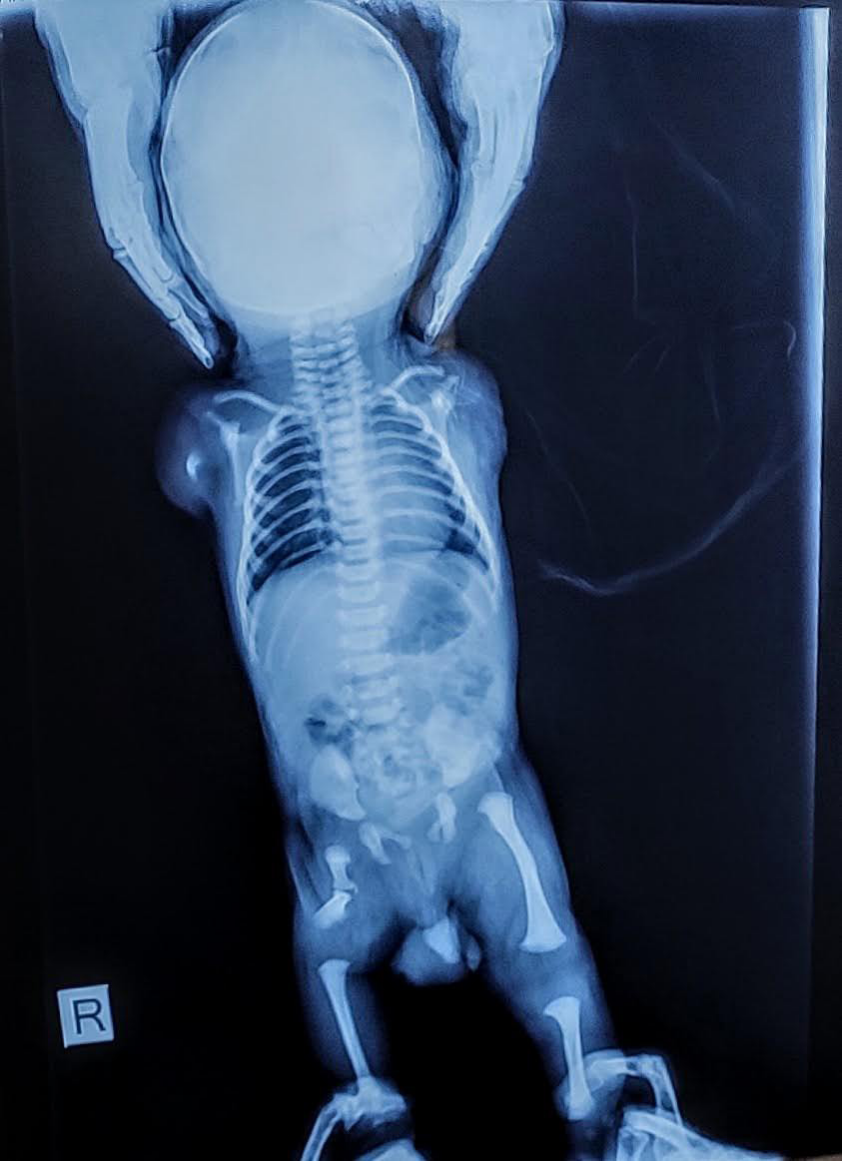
The X-ray of the baby shows a tiny bud on the right upper limb and hypoplasia of the right femur
